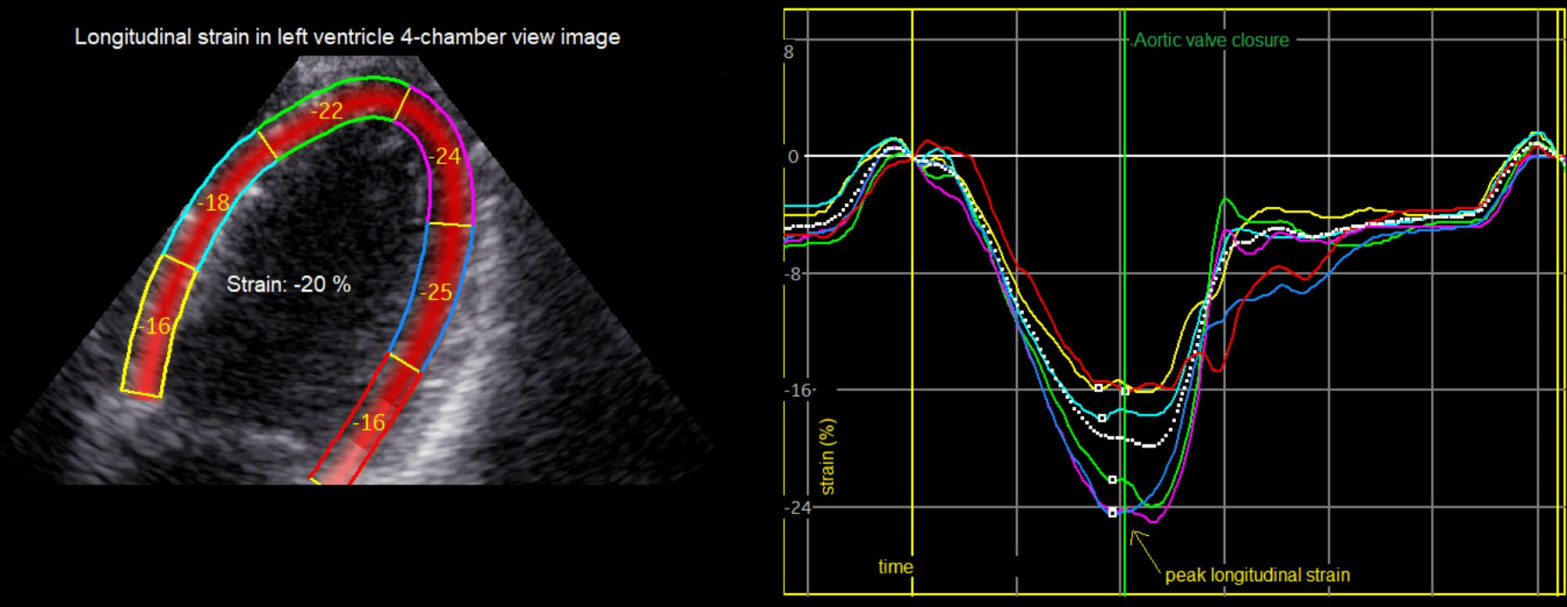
Left ventricle peak longitudinal strain measures generated from apical 4-chamber view image
